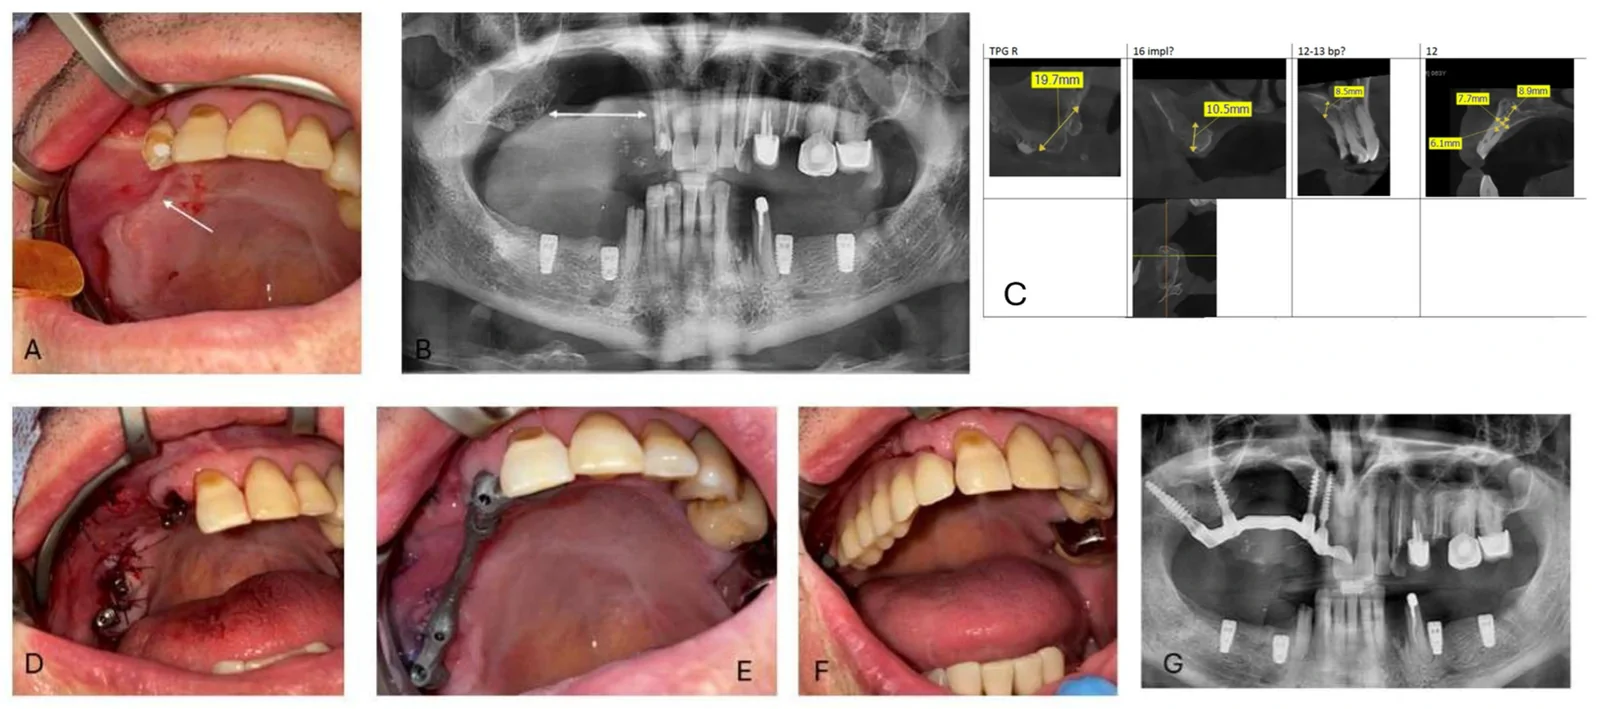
Clinical and radiographic management of Case 2. (A) Visualization of maxillary defect on the right side. (B) Panoramic X-ray on admission day. Defect of the maxilla on the right side. (C) Measurements of the height of the alveolar ridge of the upper jaw at the sites of potential implant placement. (D) Intraoral view after removal of tooth 12, placement of 4 single-piece implants and vestibuloplasty on the right side. (E) Intraoral view. Framework try-in. (F) View of the hybrid prosthesis in the mouth. (G) Post-operative panoramic X-ray with visualization of implant location and framework fitting.
